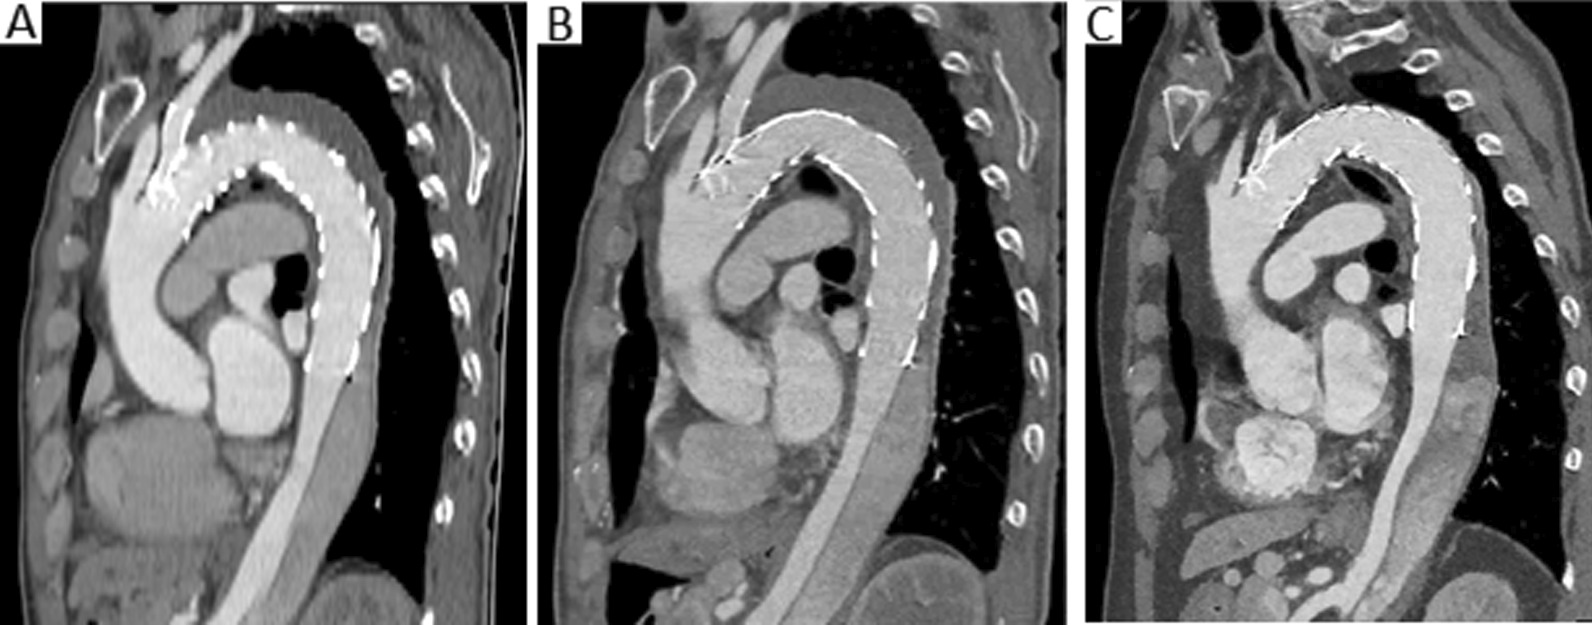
CTA on admission and during follow-up. A CTA shows persistent flow in the residual false lumen with partial thrombosis at the first admission. B CTA at readmission shows subtle progression of the thrombosis in the false lumen compared with the last admission. C CTA shows progression of the thrombosis in the false lumen without expansion at the 9-month follow-up
